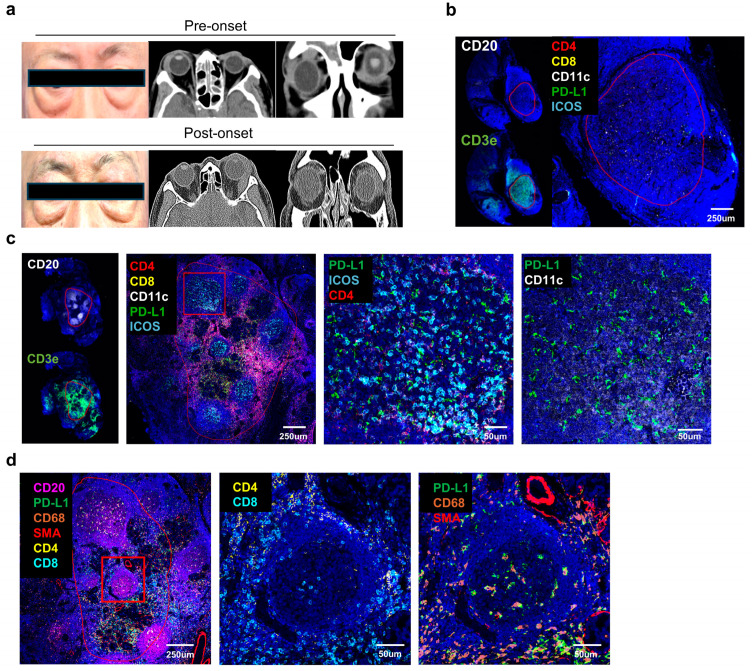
Validation in a Patient with IgG4–Related Disease Arising from Non–Specific Inflammation in the Lacrimal Gland. (a) Sixty–seven–year–old male patient with right eyelid swelling with proptosis (top). Right lacrimal gland enlargement at axial and coronary view in orbital CT (middle and right). (Bottom) Same patient demonstrated left eyelid swelling with proptosis six years later. Left lacrimal gland enlargement at axial and coronal view in orbital CT. (b) Whole image in pre–onset and (c) post–onset tissue. (d) Post-onset tissue image showing fibrotic region. Red boxes highlight fibrotic regions.
